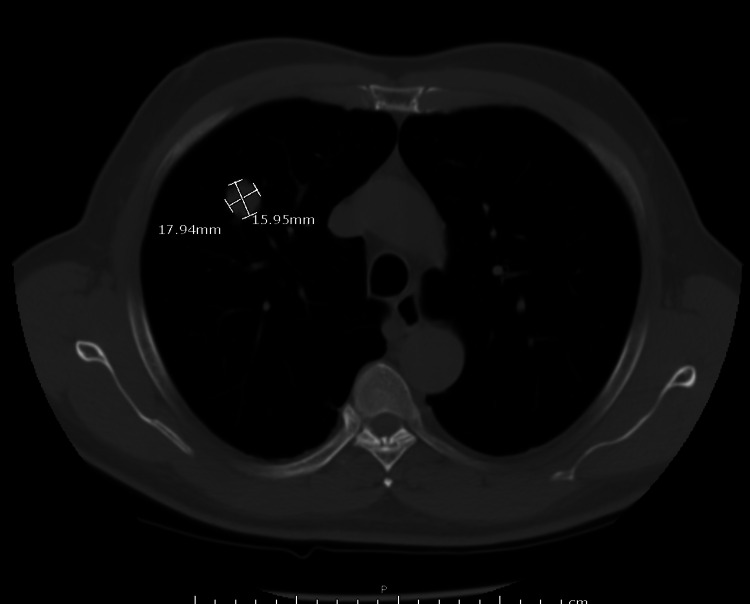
Chest CT scan confirming the presence of an RUL nodule
